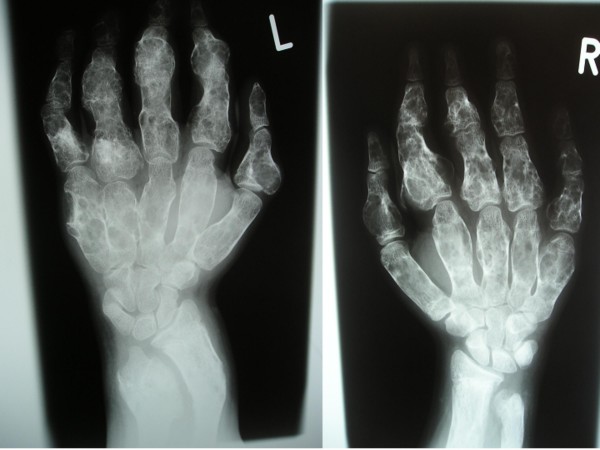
The anteroposterior hands and forearms radiograph showed irregularly expanded metaphyses and shortened diaphyses were curved over the perimetaphyseal region. Ovoid, cystic and highly radiolucent lesions, elongated parallel to the major axis of the bone, originating near the physis and migrating towards the diaphyses with growth. Shortenings, associated with bone bending causing effectively the development of Madelung's-like deformity.
